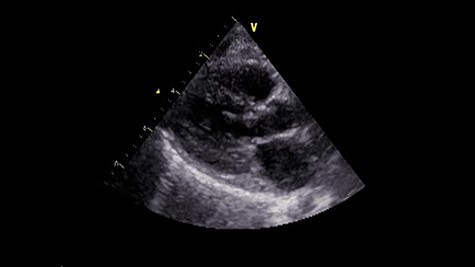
Postoperative echocardiography. The abnormal muscle band has disappeared, and the SAM of mitral valve is well controlled.
